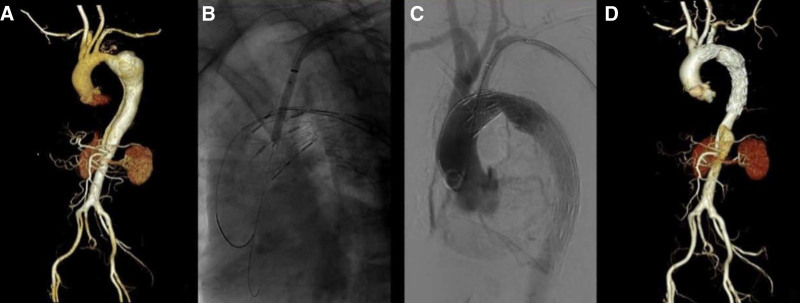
(A) CTA showed that the proximal tear of the aortic dissection was located at the greater curvature of the descending aorta, and the dissection involved the distal end of the LSA. (B, C) Intraoperative angiography showed that the stent was released accurately, the 3 branches of the arch were well developed, the tear was completely closed, the false lumen disappeared, and there was no internal fistula. (D) Follow-up CTA showed that the stent was in good position without internal fistula and the 3 branches above the arch were well developed. CTA = computed tomography angiography, LSA = left subclavian artery.
